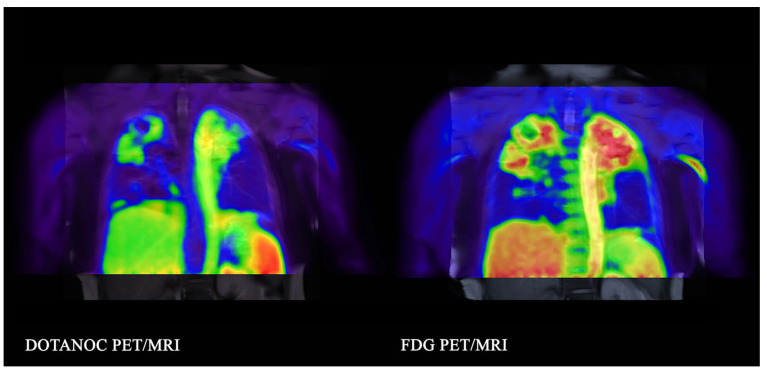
A 55 year old woman with pulmonary tuberculosis imaged with both DOTANOC and FDG PET on day 8 of treatment. Coronal images showing PET tracer uptake. DOTANOC uptake is more discrete. Reprinted unchanged with permission from ref. [21], Copyright 2020 Springer Nature.
